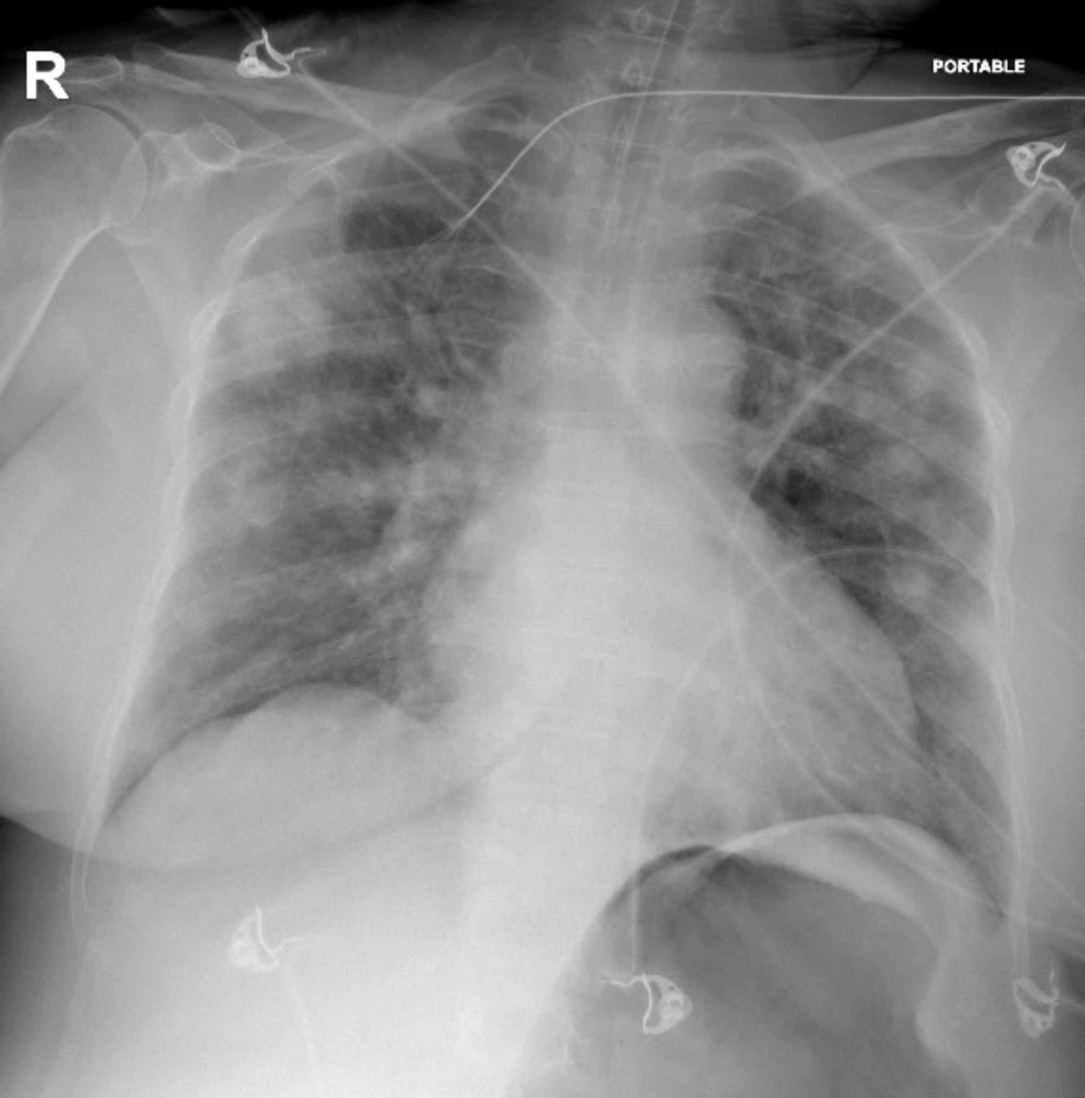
Chest X-ray shows patchy infiltrate seen bilaterally.
